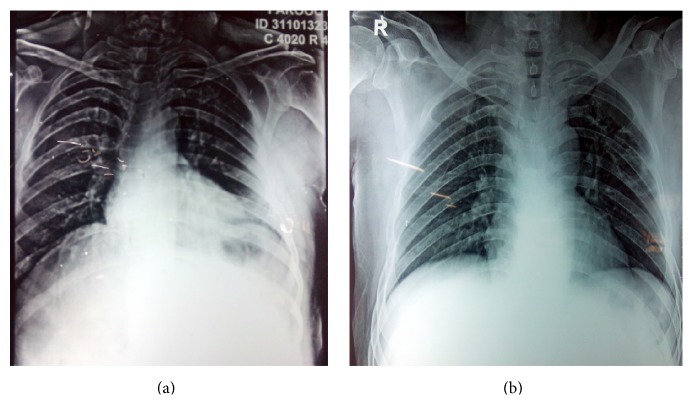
A chest X-ray on (a) showing left lower zone infiltrates. Chest X-ray on (b) at the time of discharge showing improvement.
